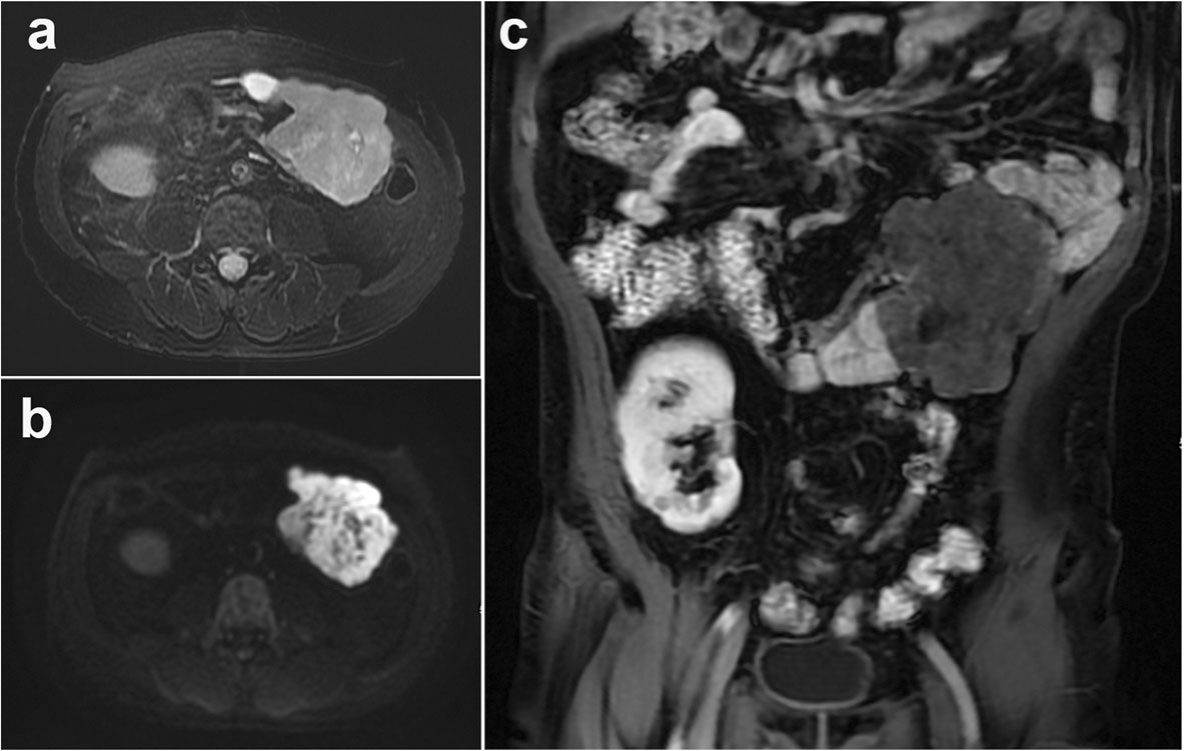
Magnetic resonance imaging scans of the gastrointestinal stromal tumor. a The tumor showed moderately high signal intensity on a fat-suppressed T2-weighted image. b The tumor clearly showed high signal intensity on a diffusion-weighted image. c Contrast-enhanced T1-weighted coronal image with fat suppression showed a weak enhancement in the tumor
